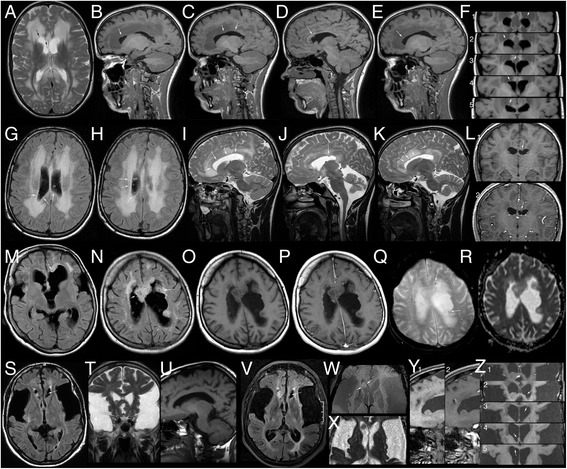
Examples of subependymal lesions. a-f MRI of *late-onset patient p1* at the age of 15.7 years with the (pre-existing) nodular subependymal lesion in the medial roof of the *right* lateral ventricle (*arrows* in a, d, f
